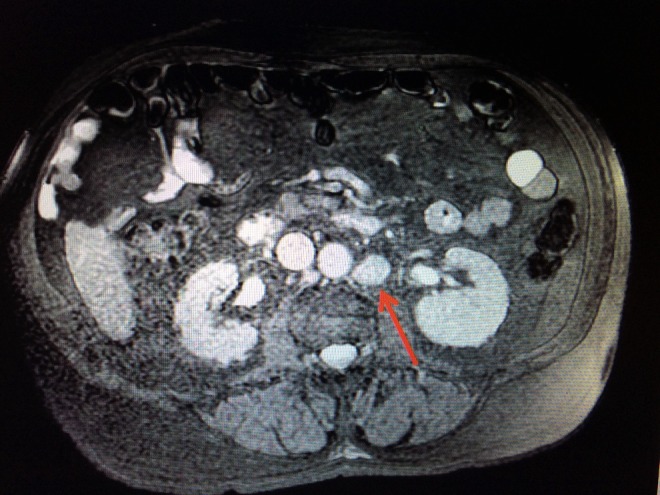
MRI of the abdomen demonstrates an enlarged 2.0 cm left para-aortic lymph node with enhancement after gadolinium (*red arrow*).
